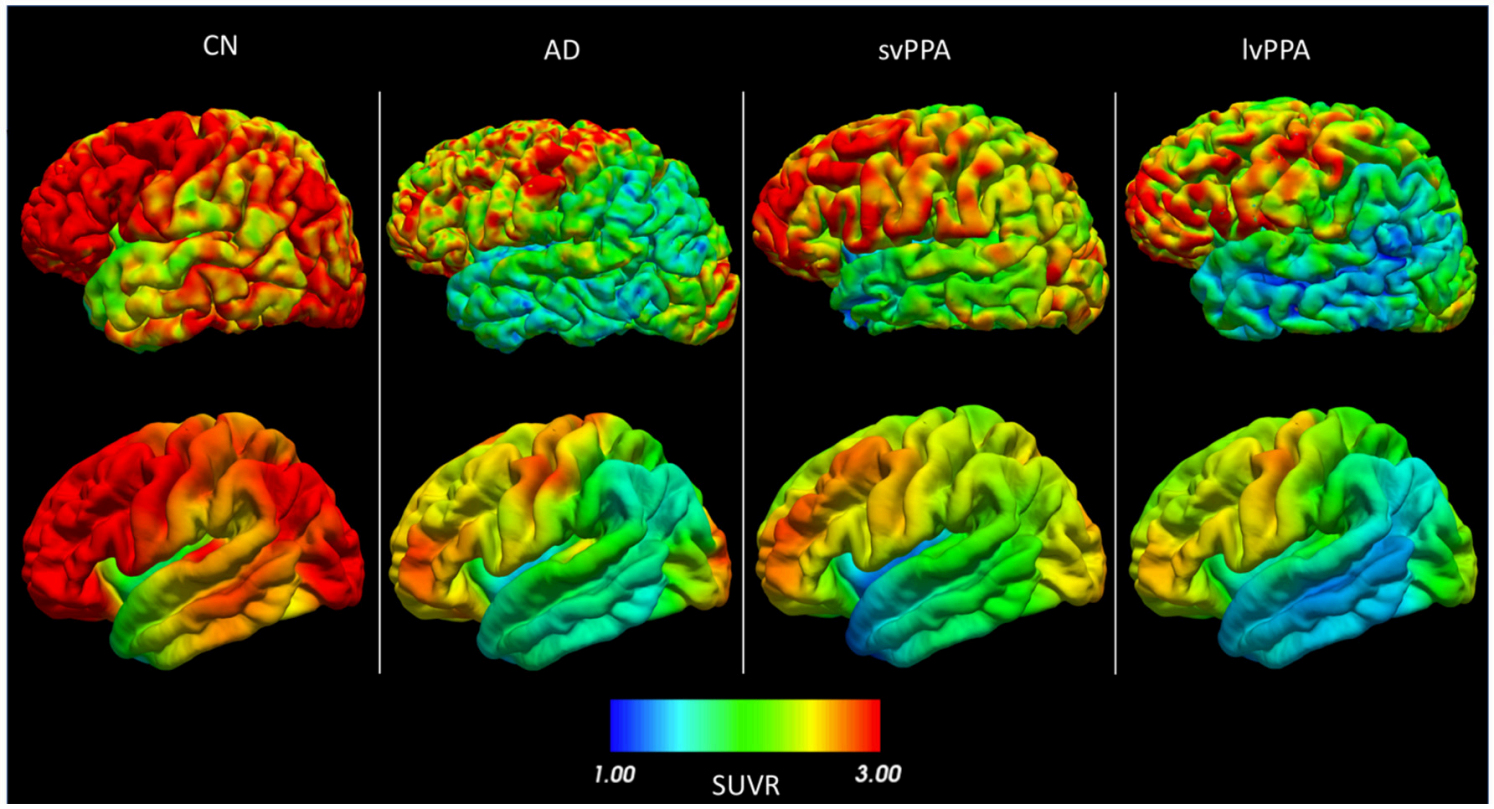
FDG PET SUVR projected onto the cortical surface (left hemisphere) for (from left to right) a cognitively normal subject (CN), a patient with Alzheimer's disease (AD), a patient with semantic variant primary progressive aphasia (svPPA), and a patient with logopenic variant primary progressive aphasia (lvPPA). The first row is the projection in the subject's space. The second row is the same signal for each subject, but warped to FsAverage after smoothing with a 20 mm Gaussian kernel.
